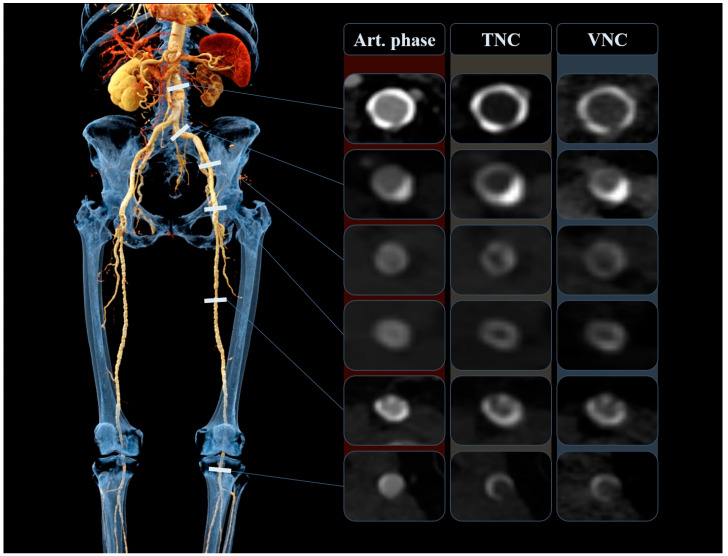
Three-dimensional cinematic volume rendered reconstruction (left) and axial image slices (right) from a representative patient, undergoing lower extremity DE-CTA. Axial slices from the arterial phase (art. phase), true non-contrast (TNC), and derived virtual non-contrast (VNC) images are shown. Quantitative measurements and qualitative analysis were conducted at six arterial levels (from top to bottom): infrarenal aorta, common iliac arteries, external iliac arteries, common femoral arteries, superficial femoral arteries, and popliteal arteries. Art. phase, arterial phase; TNC, true non-contrast; VNC, virtual non-contrast.
